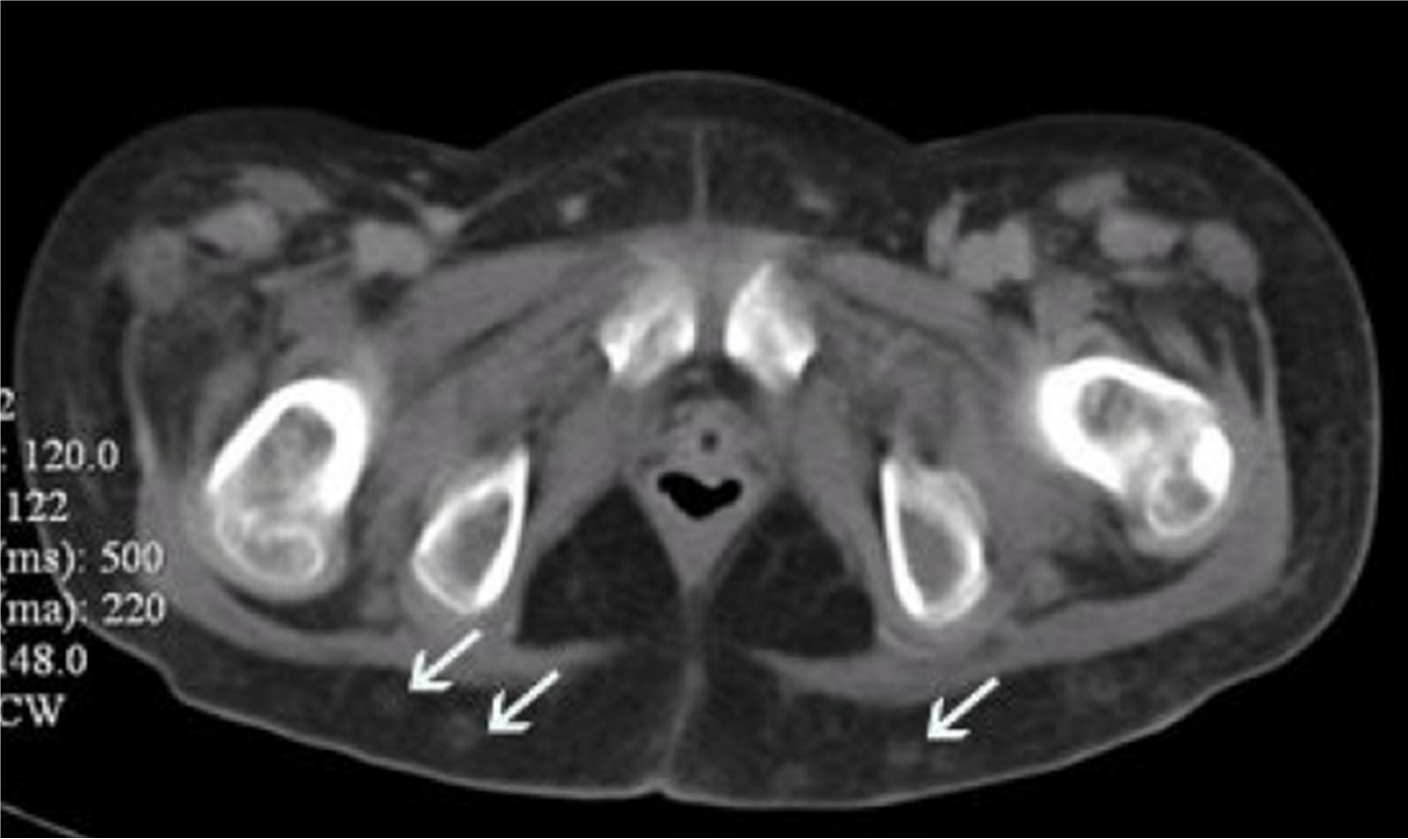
CT abdomen (transverse view). Multiple small subcutaneous abscesses(white arrows).
